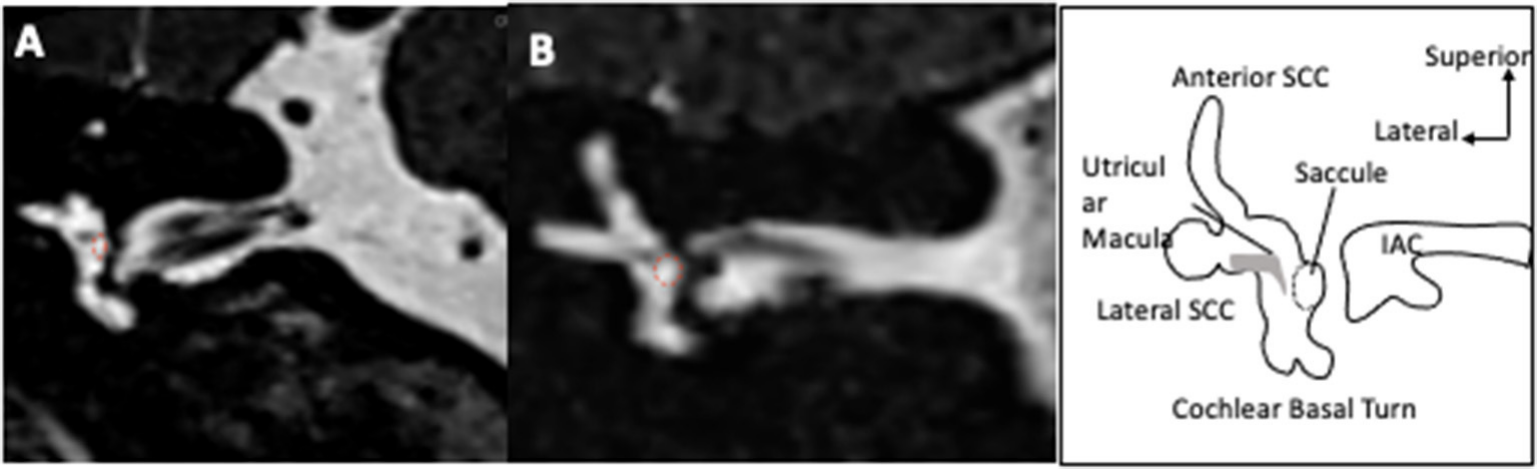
Visualization of saccule on MRI T2-weighted images. The coronal slices pass through the vestibule and show an oval shaped saccule in its superior exterior quadrant in an ear with no hydrops (A). In case of hydrops (B), the saccule is larger and approaches a circular shape. The saccule is surrounded by a dotted red circle.
